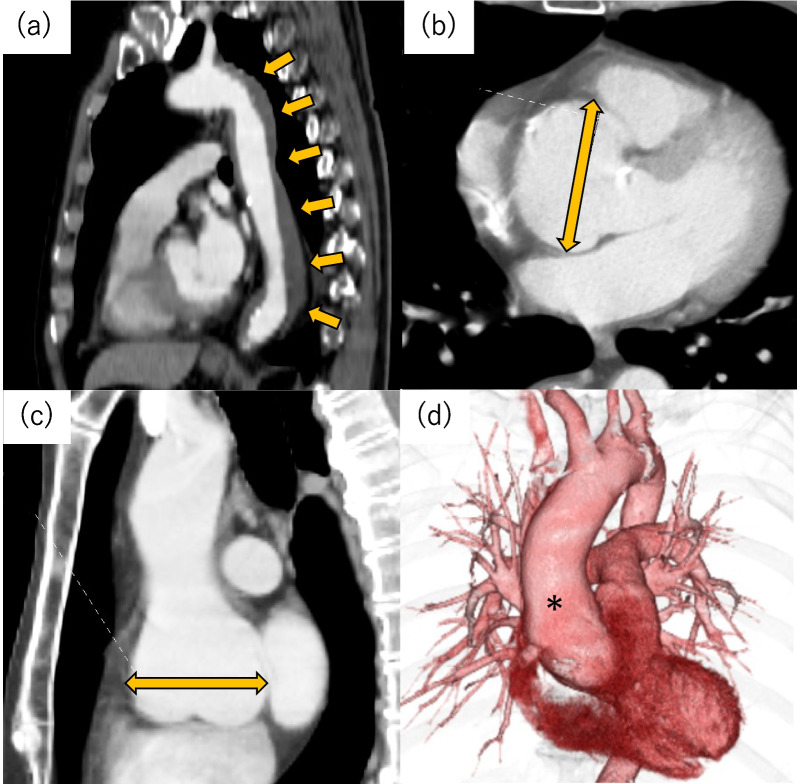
Uncomplicated Stanford type B, DeBakey type IIIb acute aortic dissection with thrombosed false lumen 14 months before surgery. Aortic root dilatation was also detected. a Stanford type B acute aortic dissection (arrows), aortic root dilatation of 55 mm in b axial and c sagittal images (arrows), d aortic root dilatation (asterisk) in 3D-CT angiography.
